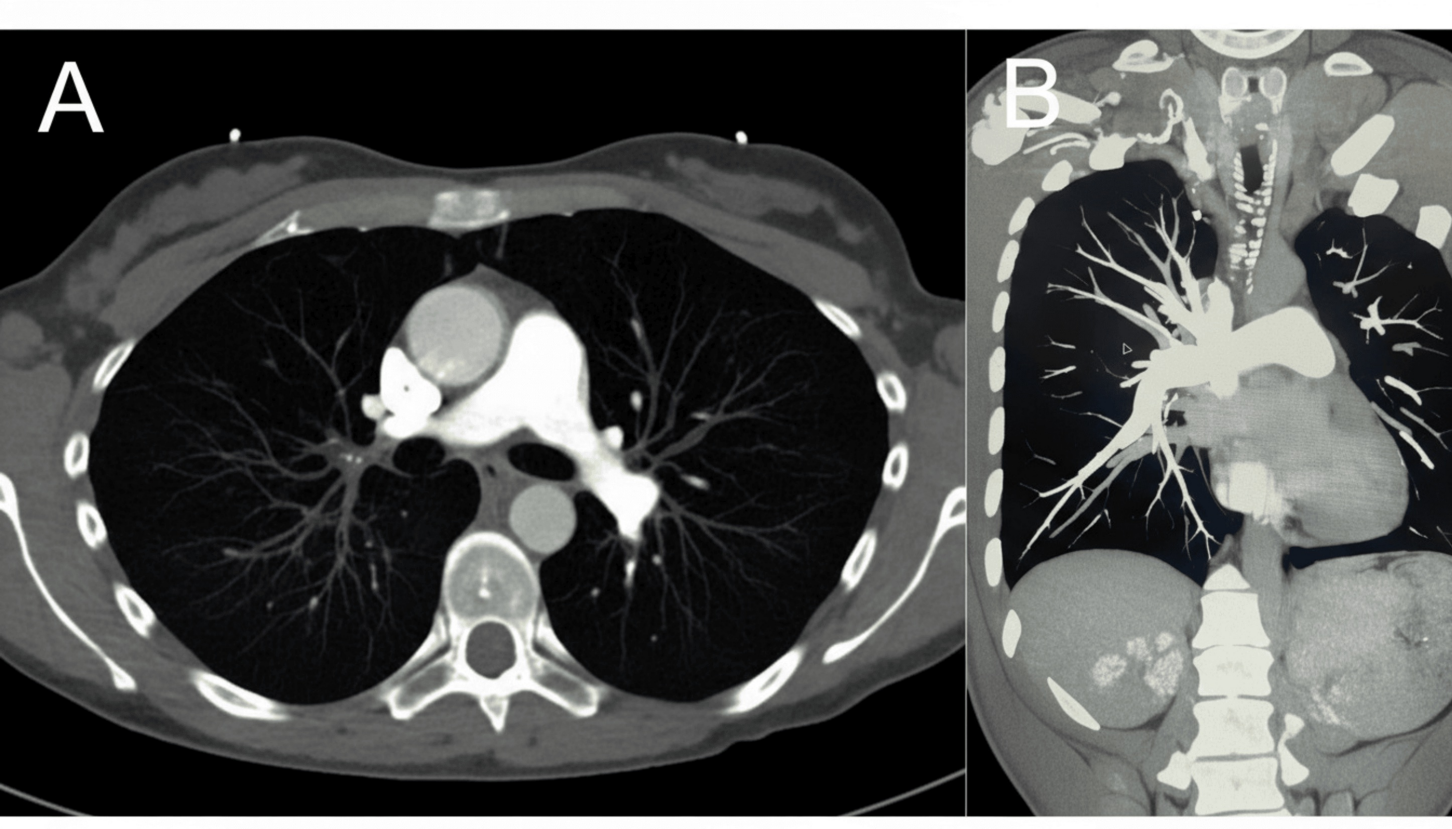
CT angiography of chest (unremarkable) (A) Axial CT pulmonary angiogram showing clear pulmonary arteries with no embolism. (B) Coronal view confirming patent vasculature and absence of interstitial lung disease. CT: computed tomography
